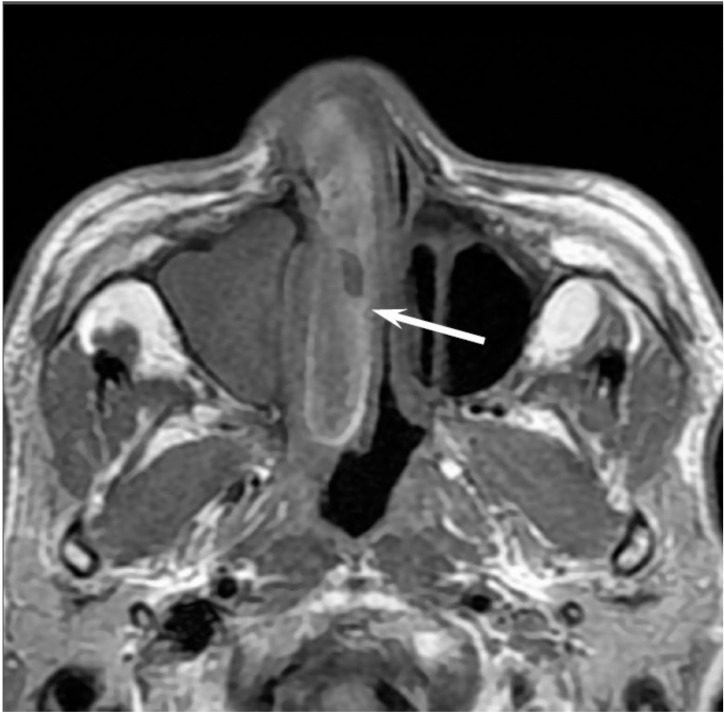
Malignant melanoma of the right nasal cavity. T1-weighted image showing heterogeneously hyperintense areas within tumor (arrow).
